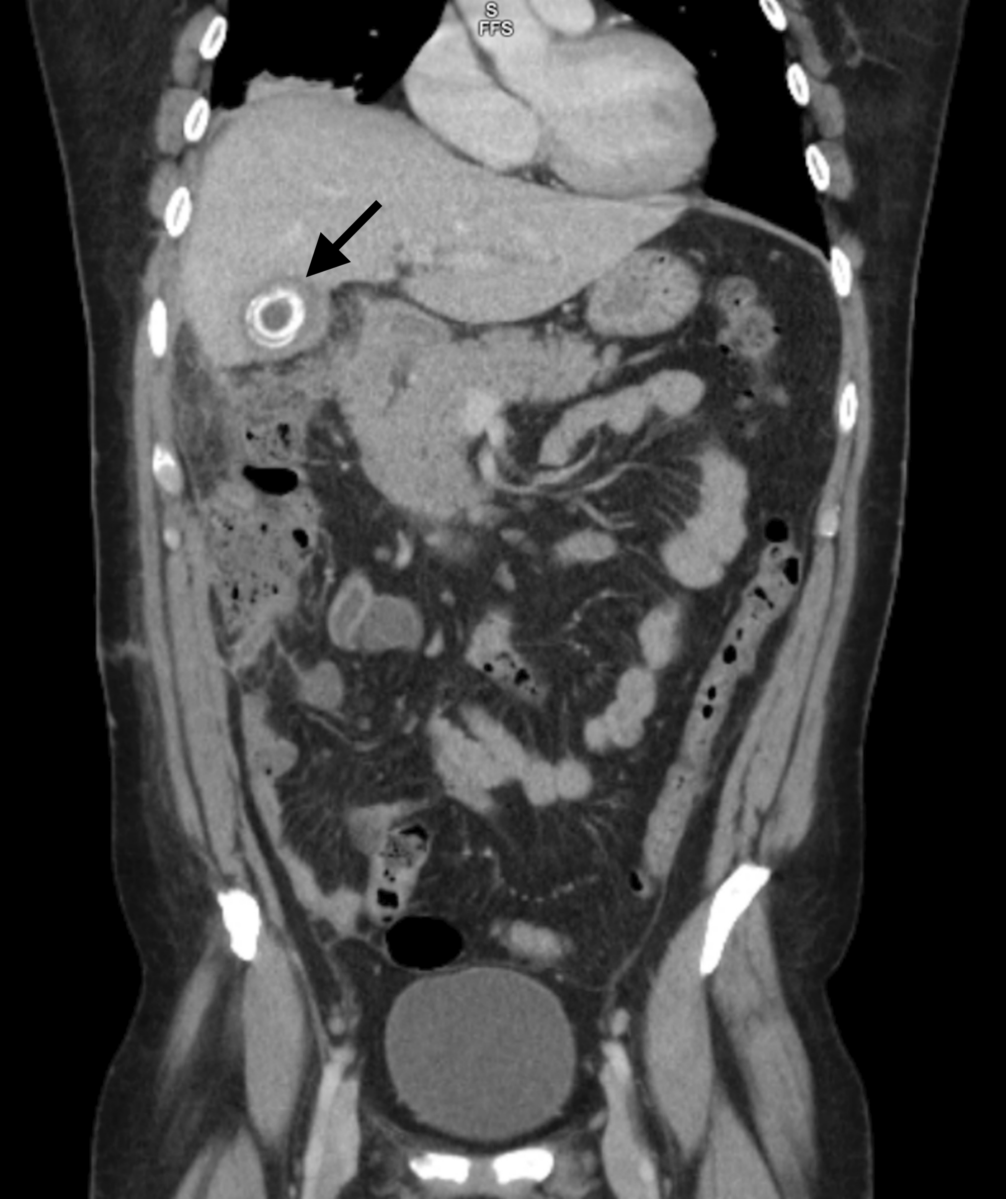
CT scan, coronal plane of calcifications within the gallbladder and surrounding inflammatory changes.
